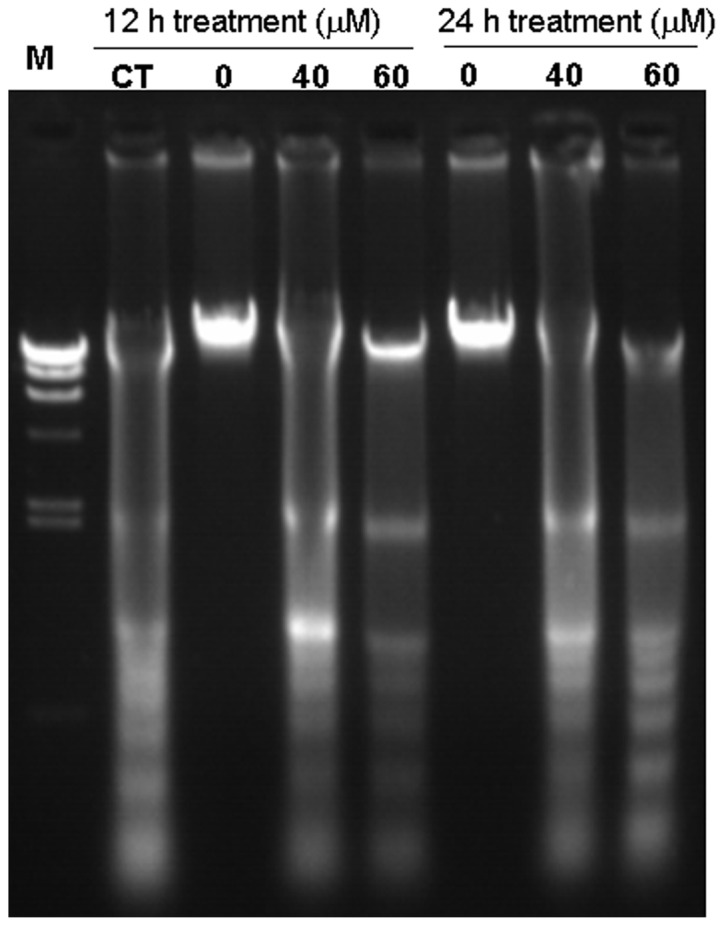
Berberine induces apoptosis-associated DNA fragmentation in MG-63 cells by the separation of urea polyacrylamide gel electrophoresis. M, marker (1 kb DNA ladder); CT, a positive control of methylnitronitrosoguanidine (20 mM).
